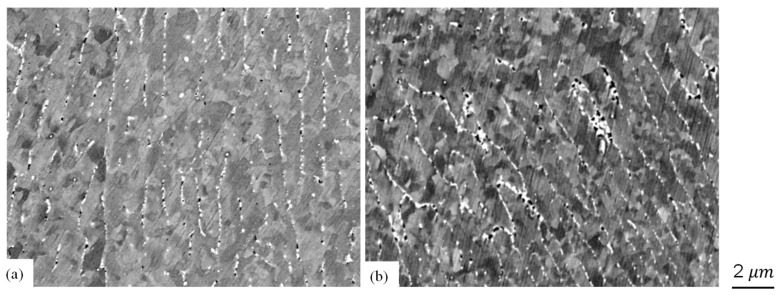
Comparison of microstructure for the case with laser power of 375 W, speed of 500 mm/s: (a) without ultrasound, (b) with ultrasound.
